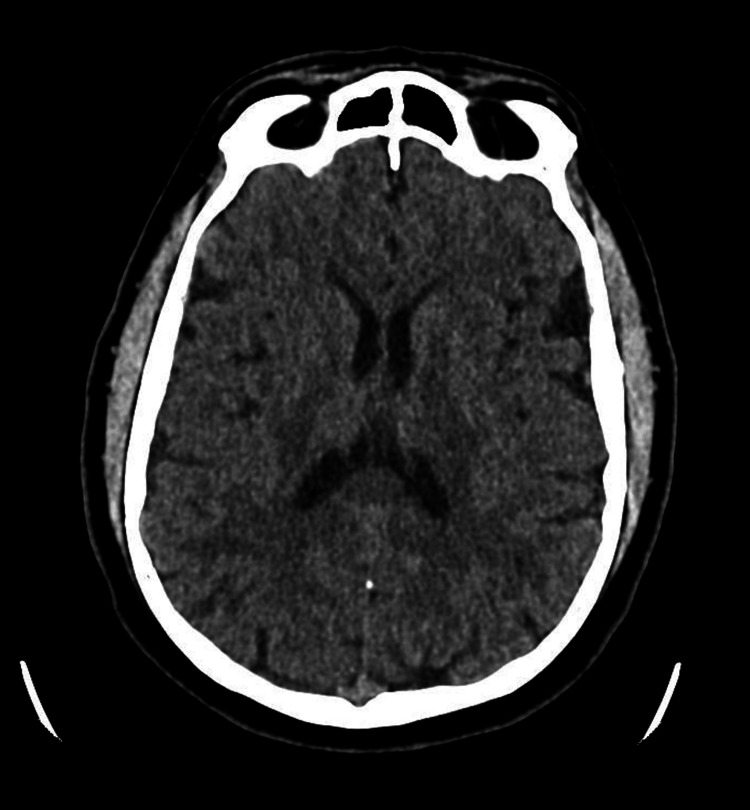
Non-contrast axial CT brain section of the patient on admission, showing no acute pathology. The lateral cerebral ventricles are normal in size, with no features of intracranial hemorrhage, infarction, or trauma.
